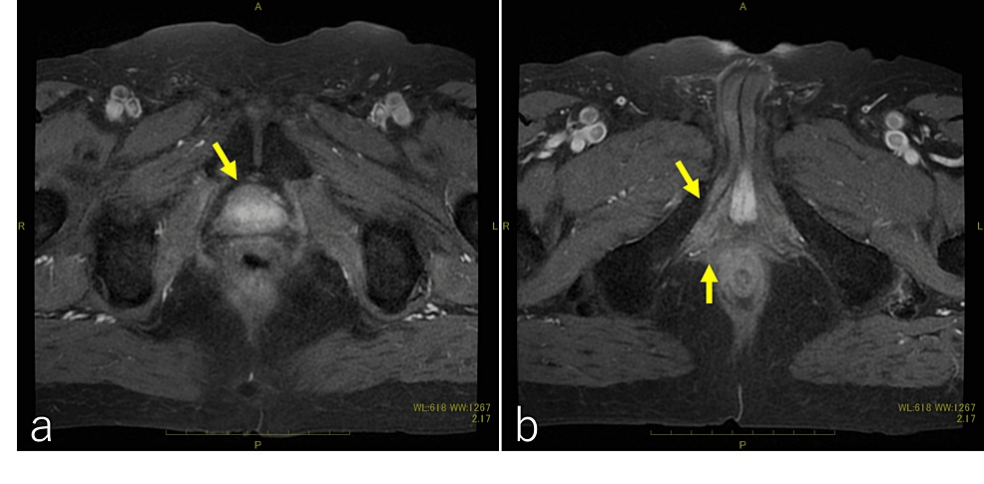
Pelvic contrast-enhanced MRI after proton beam therapy A contrast-enhancing recurrent tumor was not observed after proton beam therapy (indicated by an arrow in a); there was also no recurrence of the tumor in the intraosseous trabecularis muscle and the superficial transverse perineal muscle (indicated by arrows in b).
